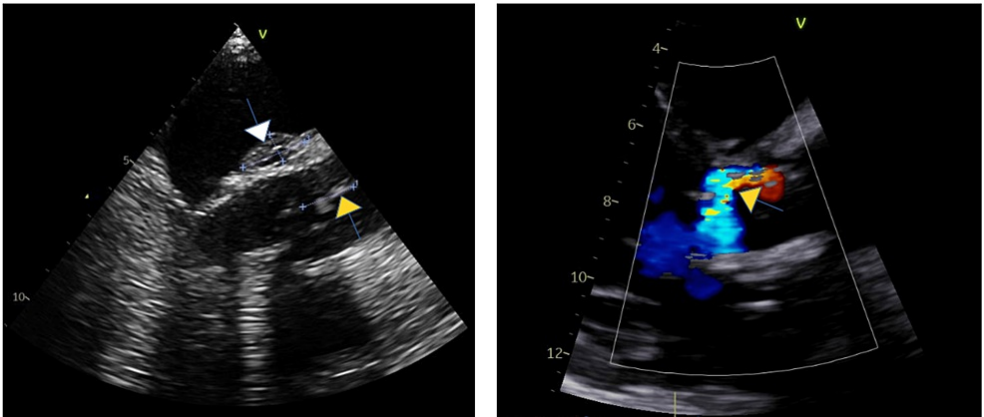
Transthoracic echocardiogram (TTE) In the left image, TTE shows a biological aortic valve with vegetation of 19 mm (yellow arrow) and an abscess on the mitral-aortic intervalvular fibrosa measuring 19x10 mm (white arrow). In the right image, TTE after biological valve replacement shows moderate intra and periprosthetic dysfunction on the aortic valve (yellow arrow).
